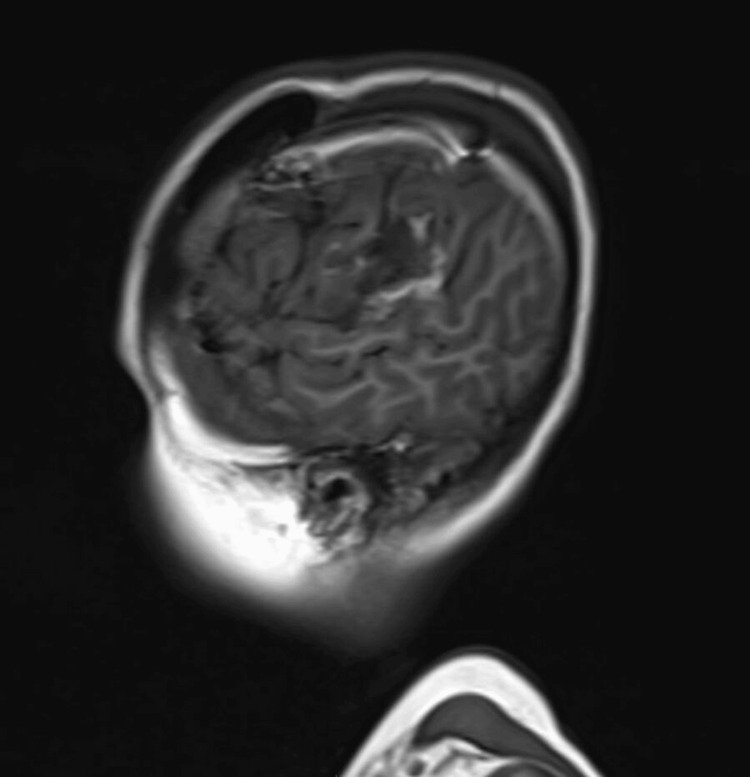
Postoperative magnetic resonance imaging scan in the T1 sagittal view The scan does not show any residual disease.
